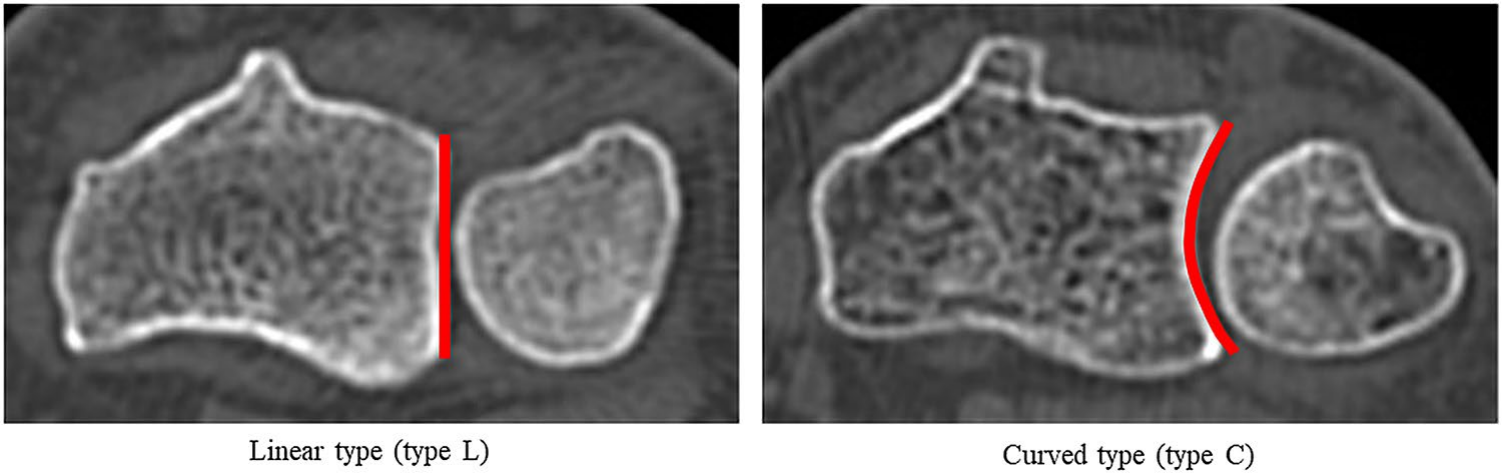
Typical computed tomographic images in the transverse plane of each type of sigmoid notch.
